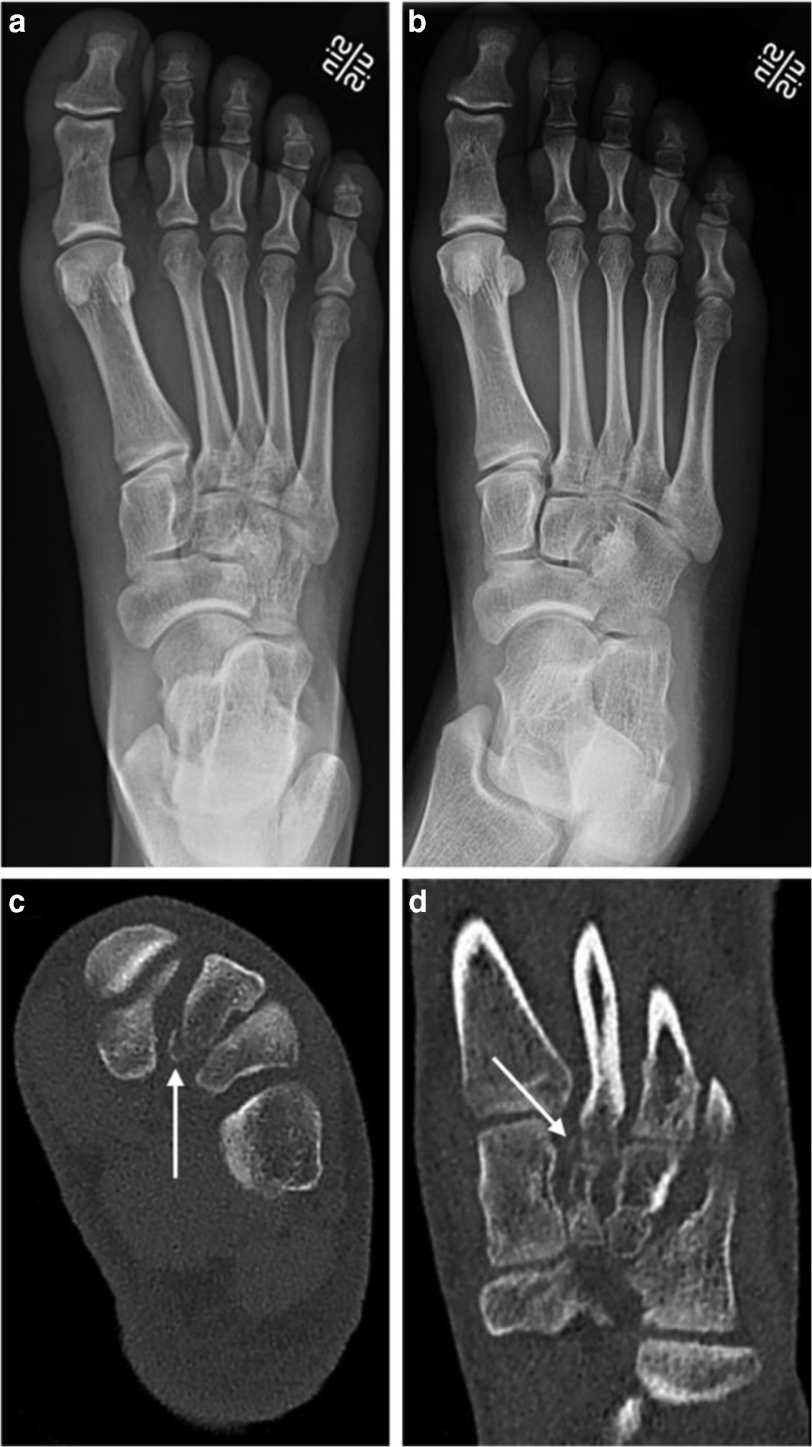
A 28-year-old female fell 2 m from a ladder and landed on her left foot. She presents to the ER unable to bear weight and with pressure pain over tarsals and base of metatarsals. DR AP and AP oblique were negative for fractures (a, b). ULD-CT demonstrates an avulsion fracture from the plantar aspect of the base of the 2nd metatarsal corresponding to the attachment of the plantar component of the Lisfranc ligament (*arrows*, c and d), making this a nondisplaced Lisfranc injury. The “fleck sign” is obvious on the oblique coronal ULD-CT image (*arrow*, d). The treatment recommendation was upgraded from functional to cast immobilization after ULD-CT
